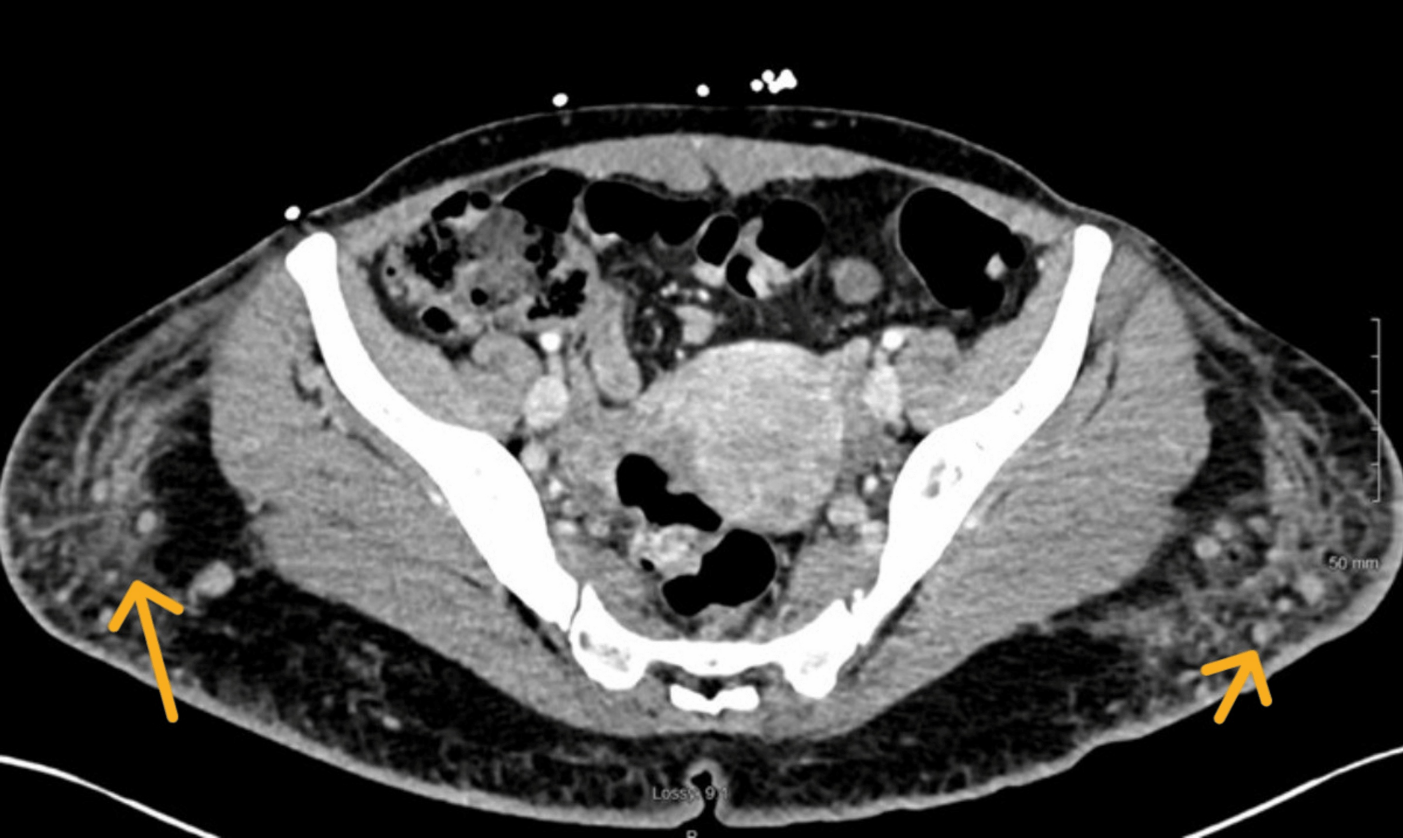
Axial CT with contrast. Yellow arrows pointing at free pellet-like findings suggestive of the injected substance.
